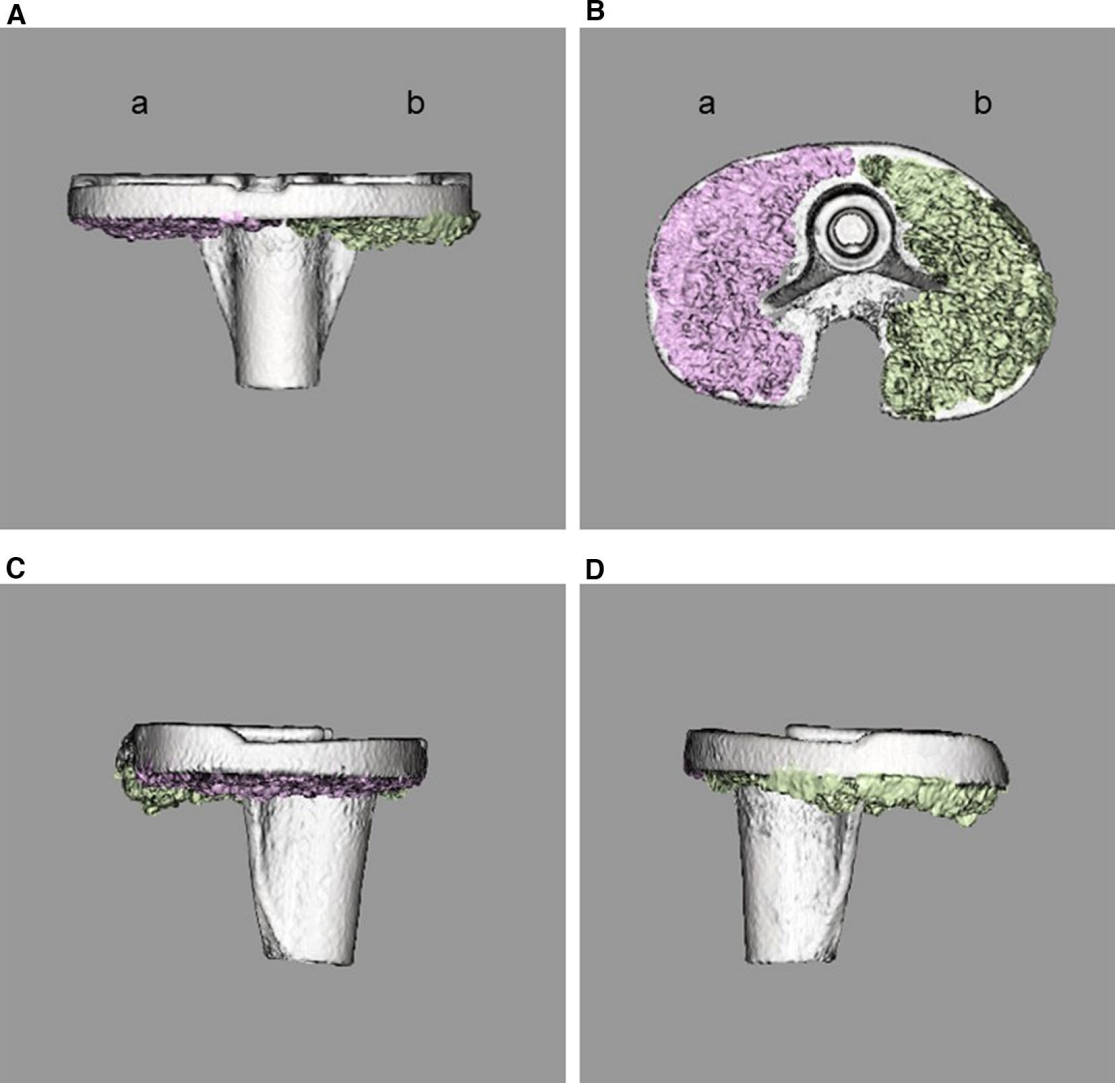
Three-dimensional computed tomography (3D CT) image of the cement penetration area. A Postoperative 3D CT frontal view. B Postoperative 3D CT caudal view. In (A) and (B), (a) lateral side and (b) the medial side are shown. C Postoperative 3D CT lateral view from lateral side. D Postoperative 3D CT lateral view from medial side. The maximum penetration in 3D CT was defined as the distance from the baseplate to the most penetrated area in each true lateral view. In any images, cement surrounding 2 mm around the keel and fin is excluded when creating the 3D CT image to prevent overestimation of cement penetration
